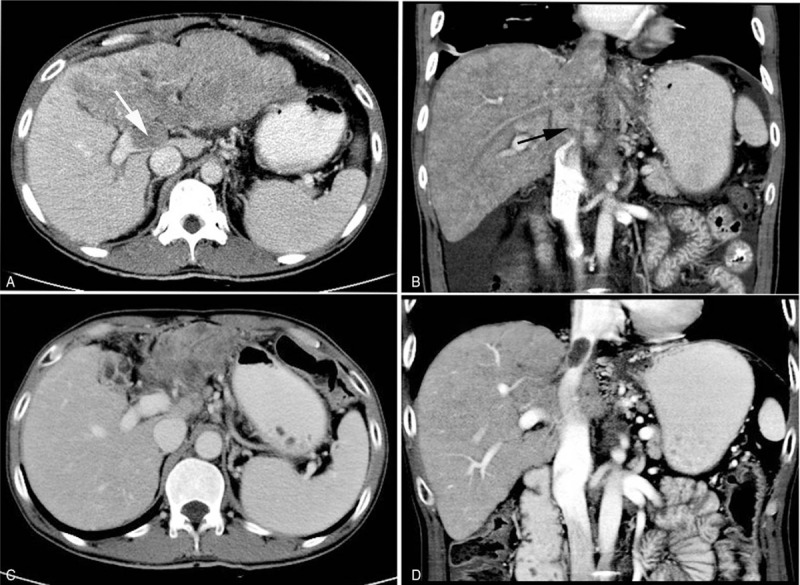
Abdomen CT images show that lesions ware located in left lobe of the liver. (A) In the venous phase, the mass is low density and irregular; the white arrow represents tumor thrombosis in portal vein. (B) The black arrow represents tumor thrombosis in inferior vena cava. (C) Intrahepatic tumors and tumor thrombosis in portal vein were diminished after oral aptinib 2 months. (D) Tumor thrombosis in inferior vena cava was diminished after 2 months.
